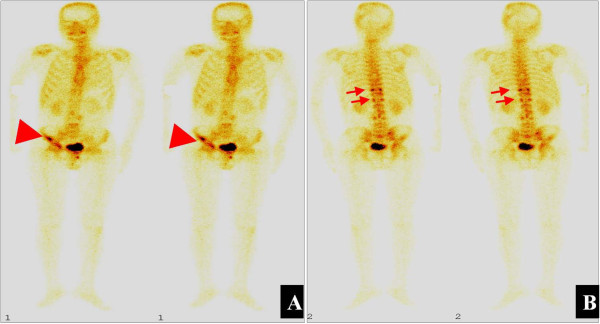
Representative emission computed tomography scans. (A) anterior scans, and (B) posterior scans, showed a hypermetabolically abnormal uptake at the right iliac bone (arrowheads) and T10, and T12 vertebral bodies (arrows).
